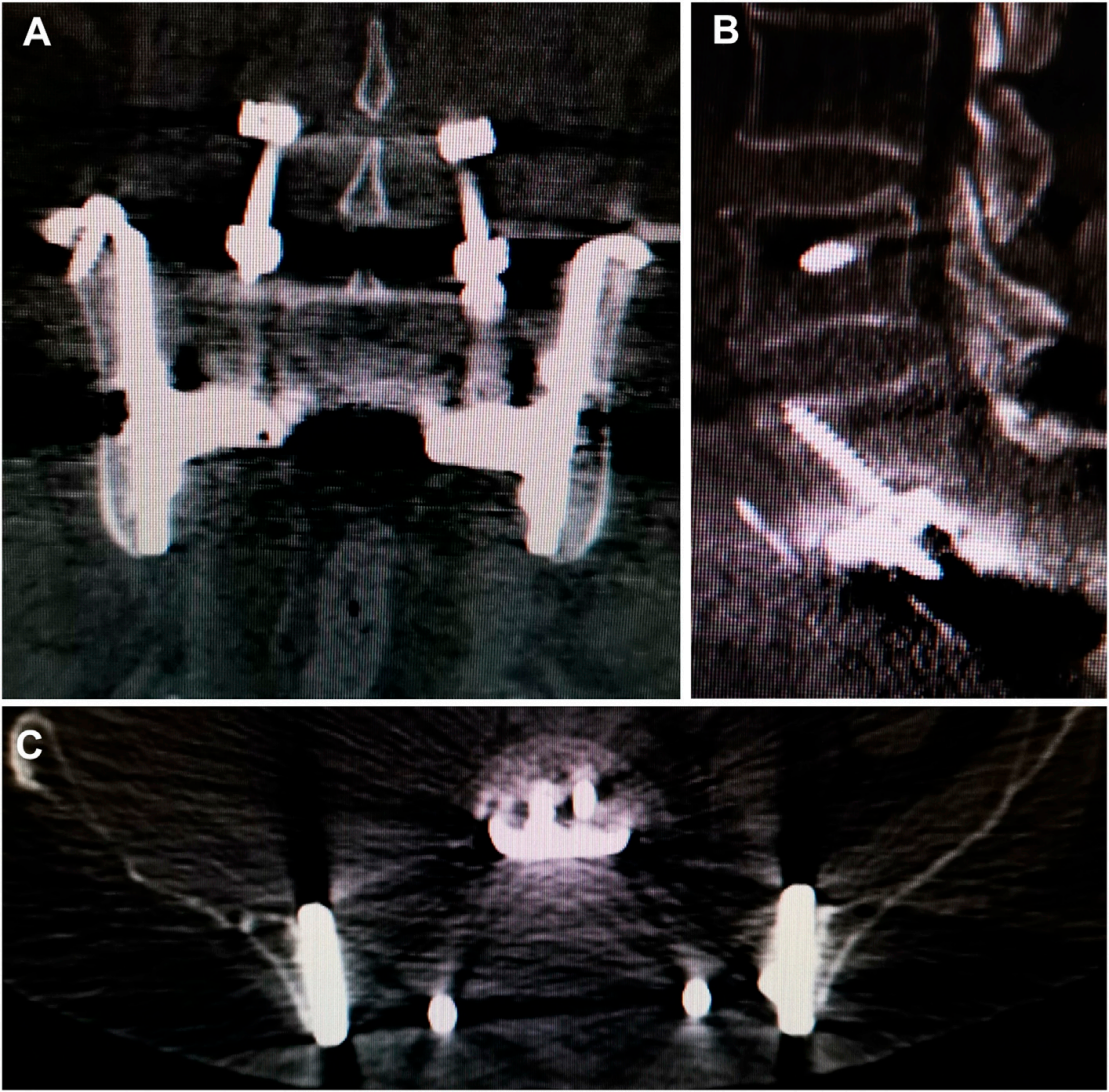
CT showed excellent osseointegration at the bone–implant junctions in coronal (A), sagittal (B). And axial (C) views.
